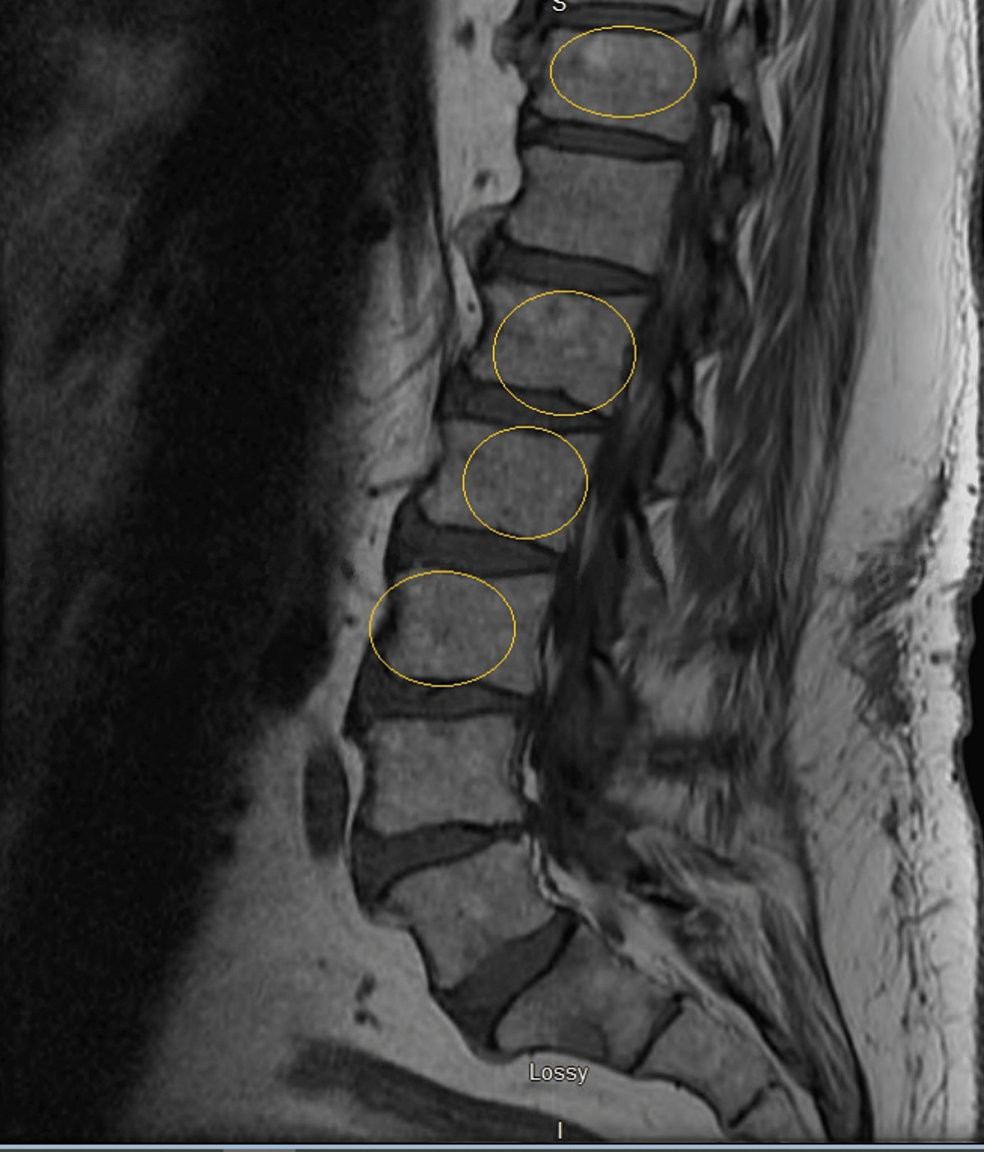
MRI of the lumbar spine revealing multiple enhancements in L1 (lumbar) and S1 (sacral) regions, indicative of metastasis.
